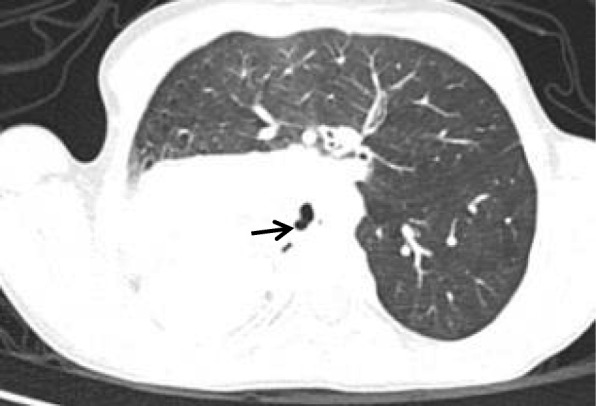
Axial lung window CT images also demonstrating rudimentary right bronchus (black arrow) with absent right lung and herniation of left lung to the right side
